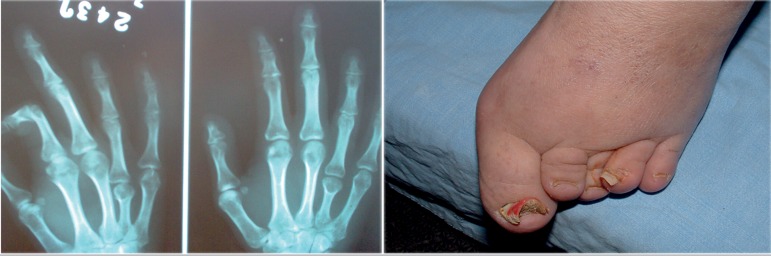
X-ray image of the hand and anomalies, patient T.A.K.: Bilateral
